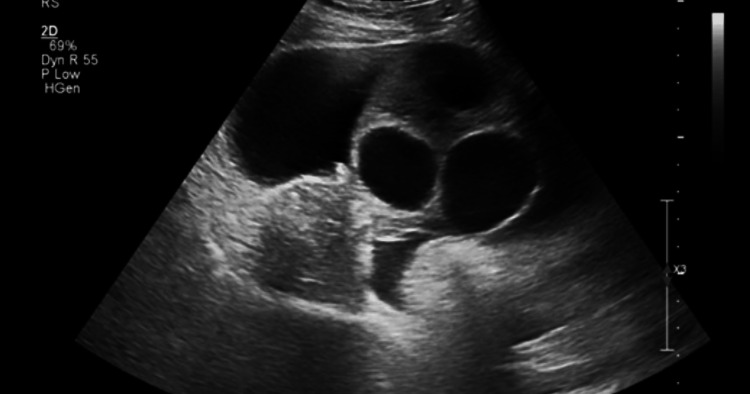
Transvaginal ultrasound showing bilaterally enlarged ovaries with multiple cystic follicles and moderate pelvic ascites.
